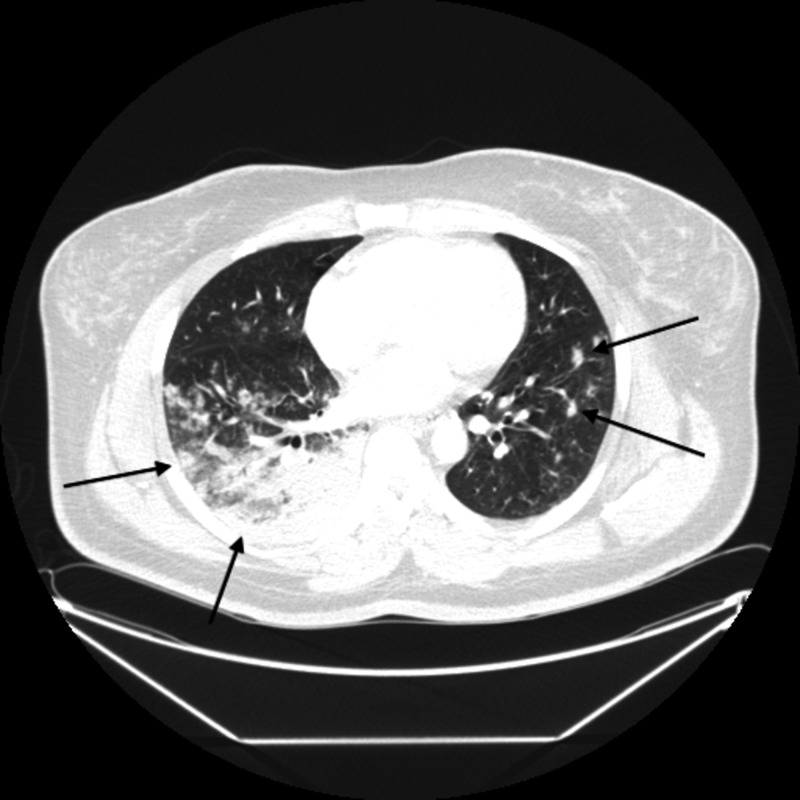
CT of the chest demonstrating bilateral consolidation, inflammation, and nodules more evident on the right side
